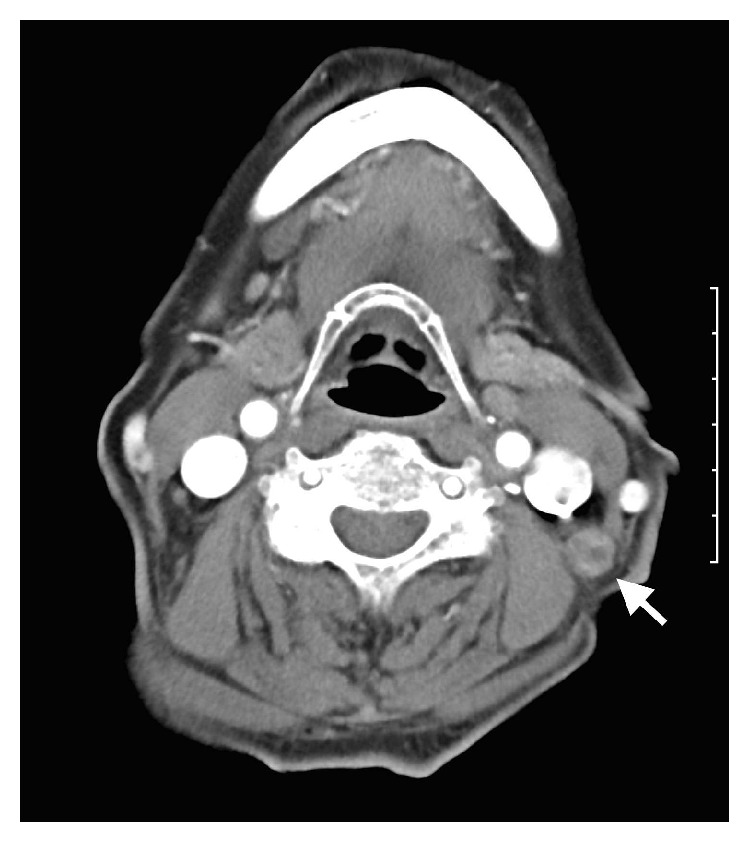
A cervical Computed Tomography scan at the initial examination showed multiple swollen cervical lymph nodes. The arrow indicates one of the swollen lymph nodes.
